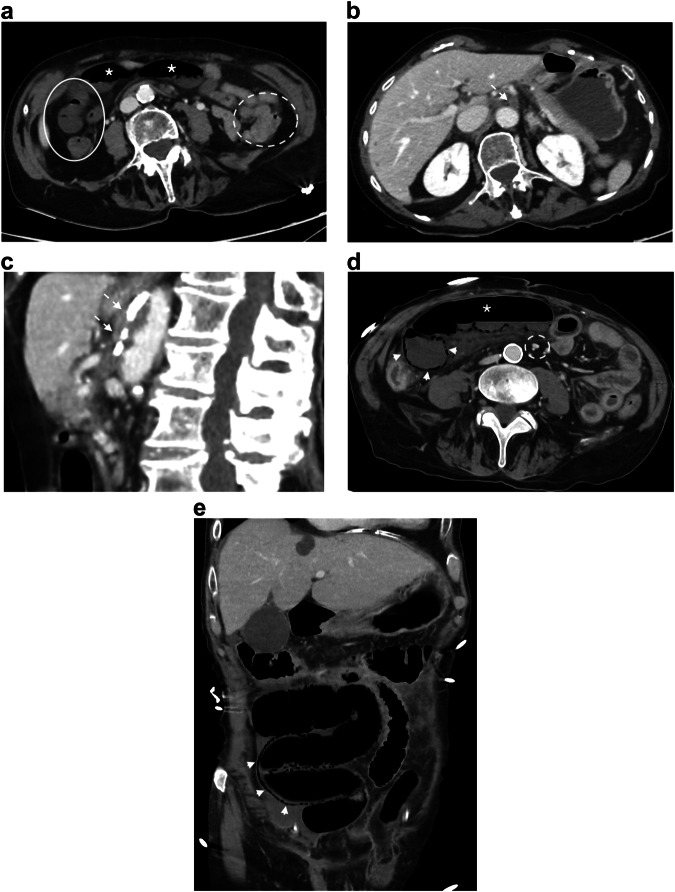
Acute mesenteric ischemia (AMI) due to mesenteric thrombosis and non-occlusive mesenteric ischemia in two different patients. AMI due to thrombosis: axial portal phase CT slice (a) in an 88-year-old woman shows decreased enhancement of the small bowel wall (plain circle) compared to another segment (dotted circle). Some of the concerned segments show a thin, “virtual” wall (asterisk). Axial portal phase CT slice at an upper level (b) shows a proximal thrombus in the superior mesenteric artery (arrow). Sagittal arterial phase CT (c) shows an occlusion of an extensively calcified mesenteric artery (arrows). AMI due to NOMI: axial and coronal portal phases (d, e) in a 78-year-old man shows dilatation of the small bowel lumen associated with a decreased enhancement of the small bowel wall and parietal pneumatosis (arrowheads). The mesenteric vessels are permeable (dotted circle)
